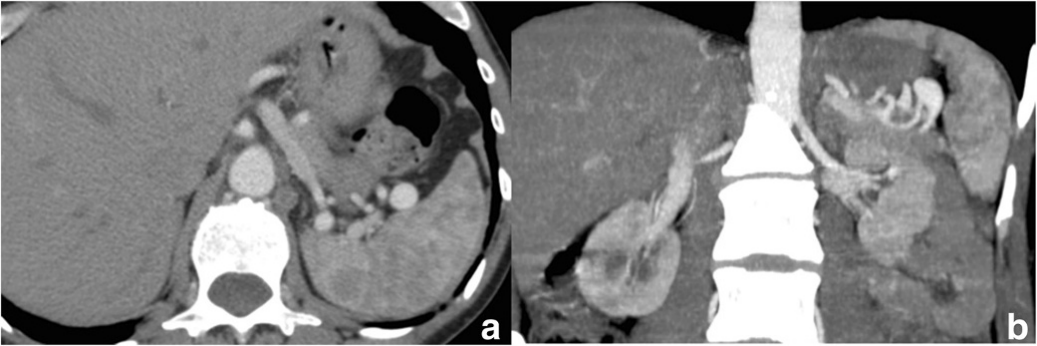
(a,b): Computed tomography scan shows splenic artery aneurysm (a); coronal view better reveals afferent and efferent vessels of the aneurismal sac (b).
